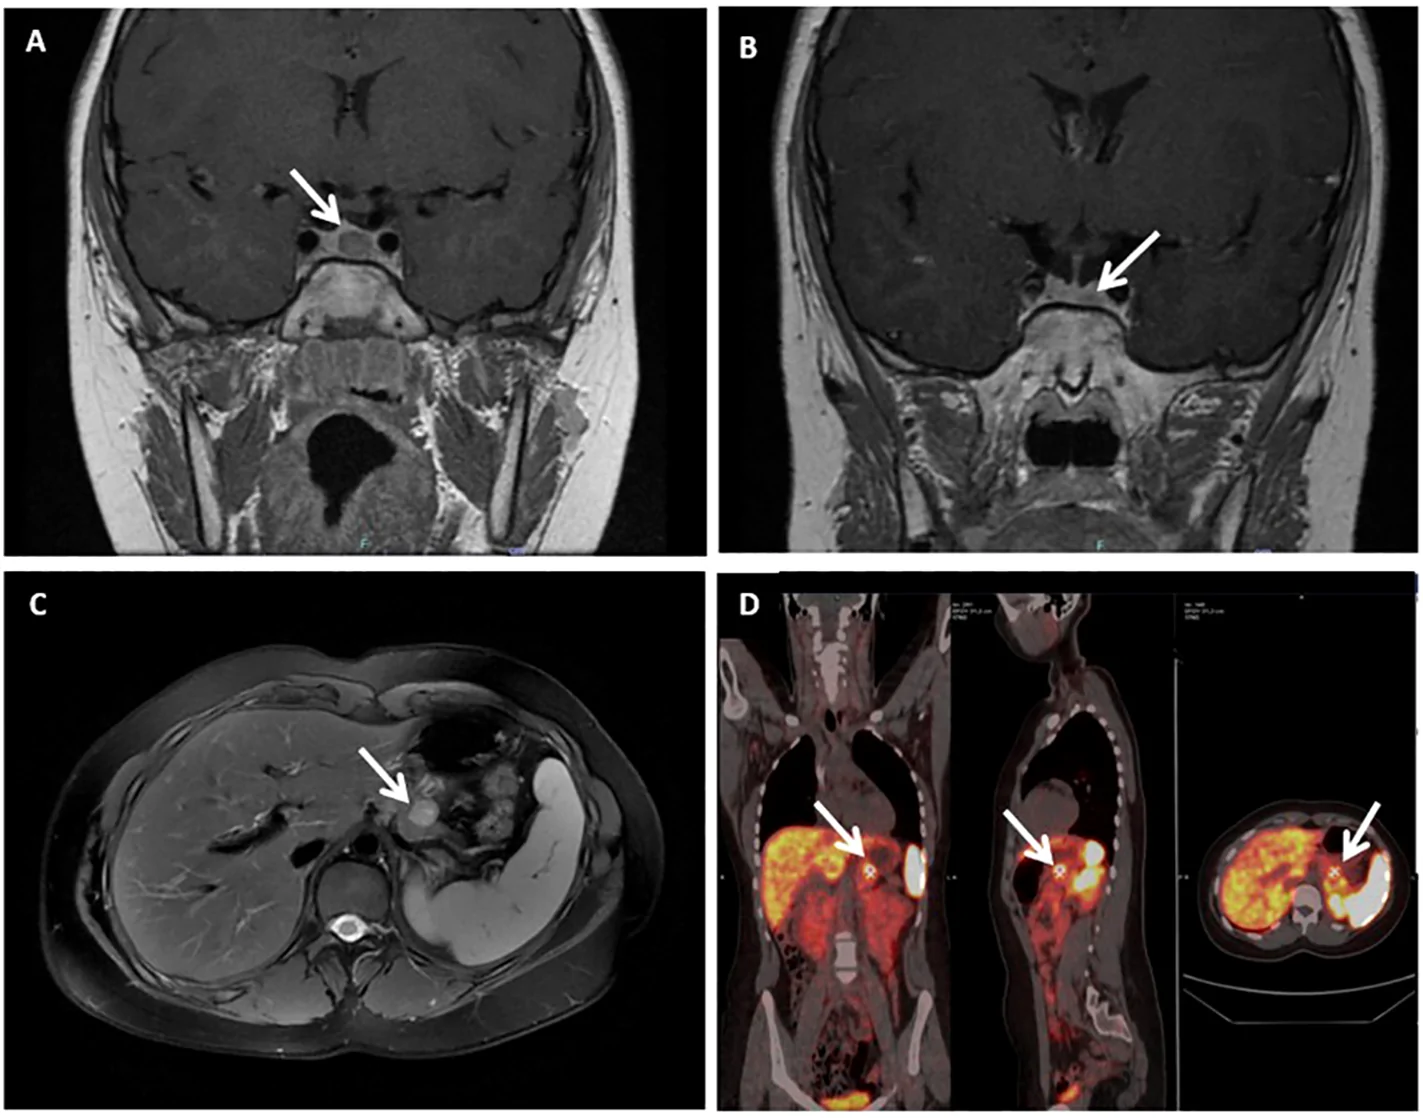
(A) T1-weighted coronal scan in Pituitary MRI of patient RG at diagnosis showing a prolactinoma localized in the anterior pituitary gland at baseline (arrow). (B) Follow-up pituitary MRI after 36 months of dopamine agonist therapy of the same patient, demonstrating significant tumor shrinkage with marked reduction in lesion size and at its original site, only a faint area of contrast hypointensity remains (arrow). (C) Pancreatic MRI of patient CS revealing a focal hypervascular lesion (16 mm) consistent with insulinoma (arrow). (D) Functional imaging (68Ga-DOTA PET/CT) of the same patient confirming increased tracer uptake in the pancreatic lesion (arrow), consistent with a well-differentiated neuroendocrine tumor.
